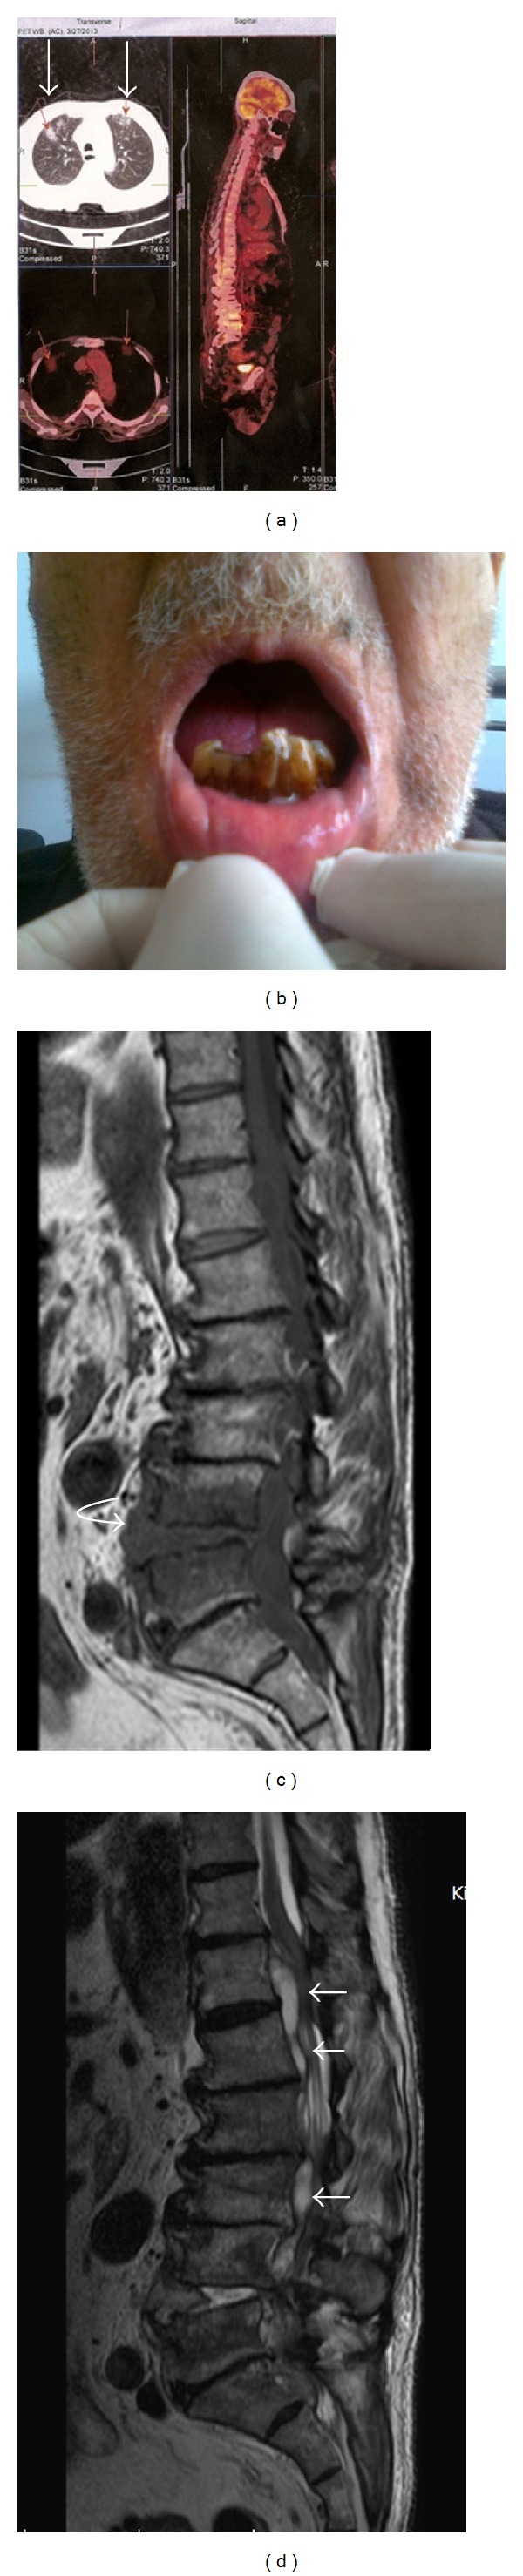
(a) PET-CT image of the patient showing pulmonary and lumbar vertebral involvement (arrows). (b) Poor orodental hygiene of the patient of heavy smoking hand-rolled tobacco. (c) T1 weighed sagittal MRI of the spine showing edema and postcontrast involvement in L4-5 vertebral bodies and postcontrast uptake L4-5 intervertebral disc (arrow) and adjacent iliopsoas muscle (not shown in this image). (d) T2 weighed sagittal MRI reveals epidural fluid with an 8 mm width at most between T12 and S1 vertebrae, in an unconnected extension with peripheral contrast involvement. There are a marked spinal cord and neural foraminal compression (arrows).* Actinomyces israelii *was isolated from the culture of the abscess and disc material.
